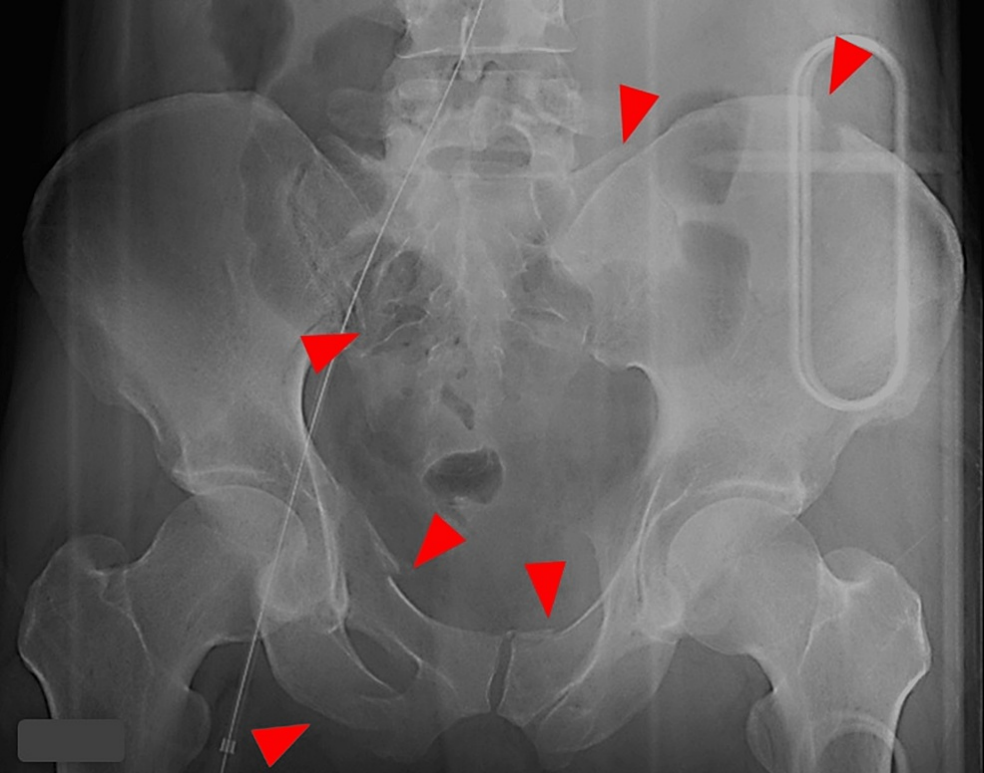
Pelvis X-ray. Multiple fractures (arrowheads) are found on both sides of the pelvis on the X-ray.
